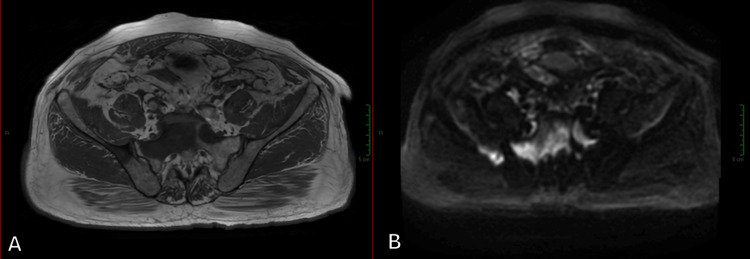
Bone metastases affecting the sacrum and right iliac body and wing, appearing as hypointense areas on T1WI with associated restricted diffusion on DWI (A) Axial TWI. (B) Axial DWI. DWI, diffusion-weighted imaging; T1WI, T1-weighted imaging
